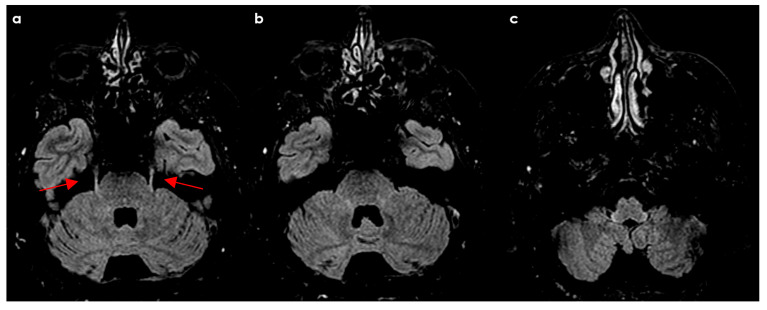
Six-month follow-up MRI of the same patients as in Figure 1, Figure 2 and Figure 3, showing further improvement of the findings with persisting linear hyperintensity along the TN course (red arrows) in ascending axial FLAIR slices from (a–c).
